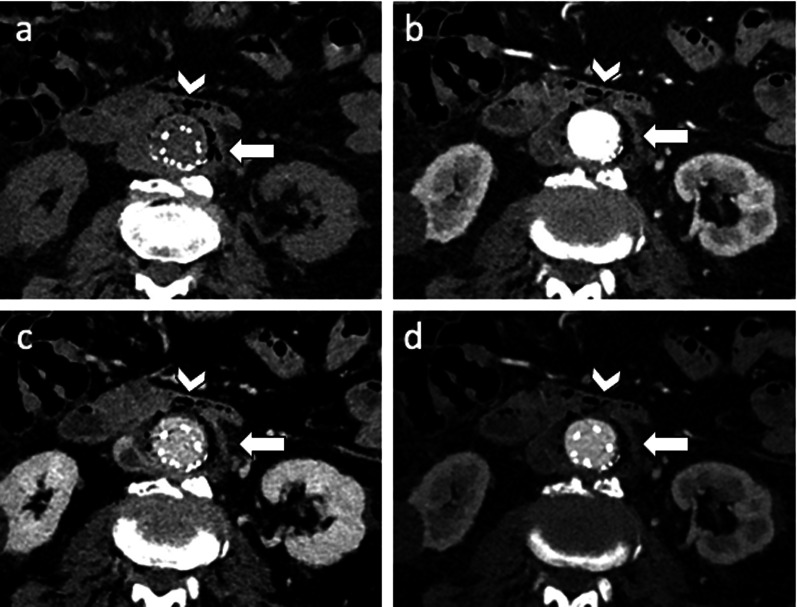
81-year-old man with aorto-enteric fistula. Axial CT images on (a) pre-contrast, (b) arterial, (c) portal and (d) delayed phases show small air bubbles around the graft (arrow) and communication between the second portion of duodenum and the aneurysm sac as an aortoduodenal fistula
